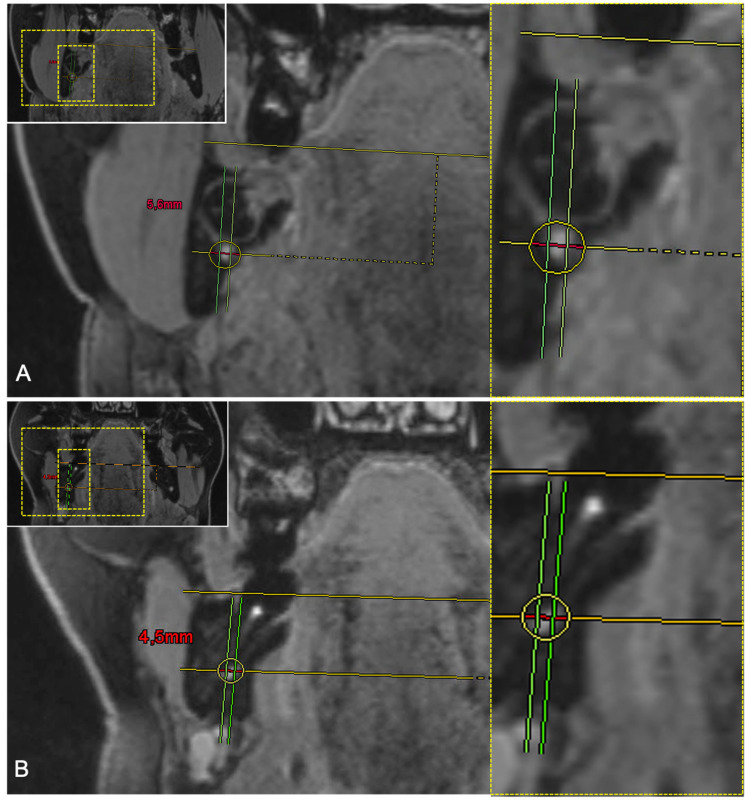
(A) Coronal reconstruction of a study participant’s 3D-DESS MRI images visualizing the intraosseous IAN evaluation at the third molar. For orientation, the dotted rectangles in the corner show the enlarged area. The upper middle segment (segment 2) and the lower middle segment (segment 5) are occupied by MRI signal hyperintensities. (B) Coronal reconstruction of a study participant’s 3D-DESS MRI images visualizing the intraosseous IAN evaluation at the level of the second molar. The upper lingual segment (segment 3), lower buccal segment (segment 4), and the lower middle segment (segment 5) are occupied by MRI signal hyperintensities.
